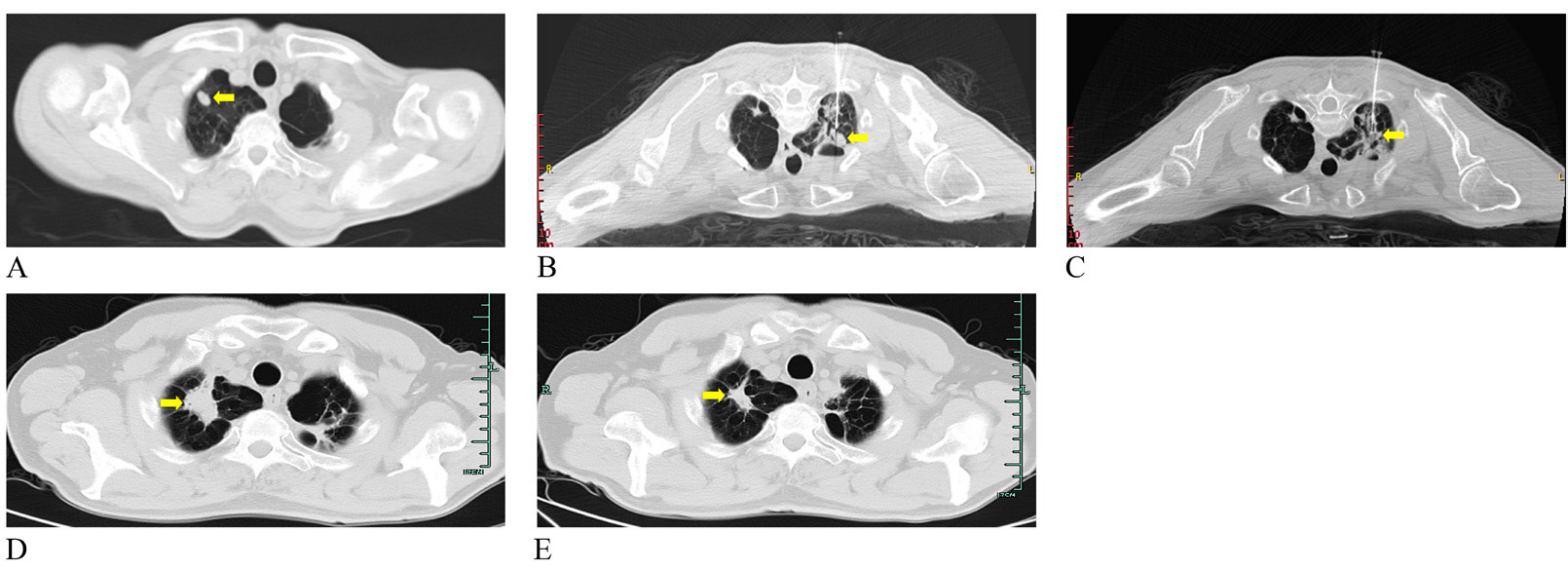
Representative CT images from a 62-year-old man diagnosed with lung metastasis from liver cancer A. Preoperative unenhanced CT image before PLA shows tumor about 14.5*7.7 mm in the right lung (yellow arrow). Intraoperative CT image shows the arranging needle method of using two laser fibers parallelly ablating the tumor (B. Before ablation; C. After ablation) (yellow arrows). Postoperative CT image in axial plane indicated the increased high-density lesion that has been ablated two months later D. and reduced in four-month follow-up E. (yellow arrows).
